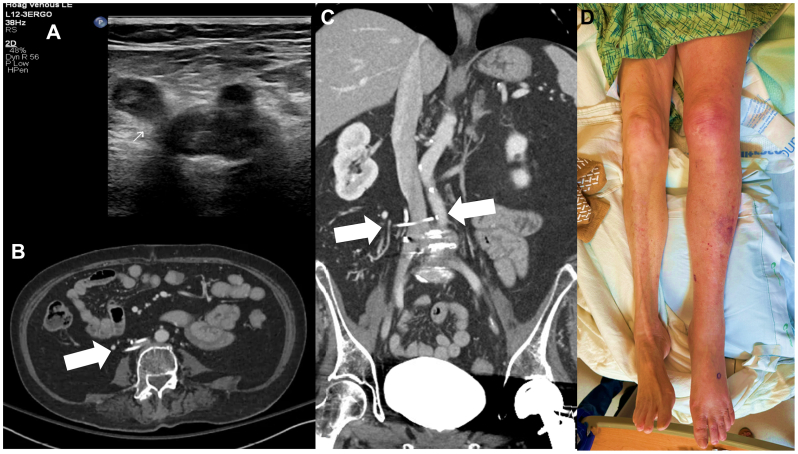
The patient presented with an acutely discolored and swollen left lower extremity with progressive paresthesia. (A) Venous ultrasound examination demonstrates occlusive thrombus throughout the left lower extremity, including at the saphenofemoral junction, as shown here. (B) Computed tomography (CT) venogram axial image demonstrates left lower extremity thrombosis (not shown) and also reveals an Moretz inferior vena cava (IVC) clip encircling the lower IVC (*white arrow*). (C) CT venogram coronal reformat shows again the IVC clip in place (*white arrows*). (D) Picture of patient legs showing markedly swollen and taut left lower extremity with purpuric discoloration. Bedside evaluation revealed nonpalpable left pedal pulses, whereas right pedal pulses were easily palpable. Left pedal pulses are audible only on Doppler ultrasound examination.
